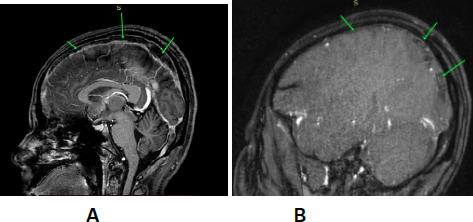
MRI of the brain with MRV A) showing loss of normal blood flow in the entire superior sagittal sinus, B) acute thrombosis with venous infarction of bilateral frontal lobes.
